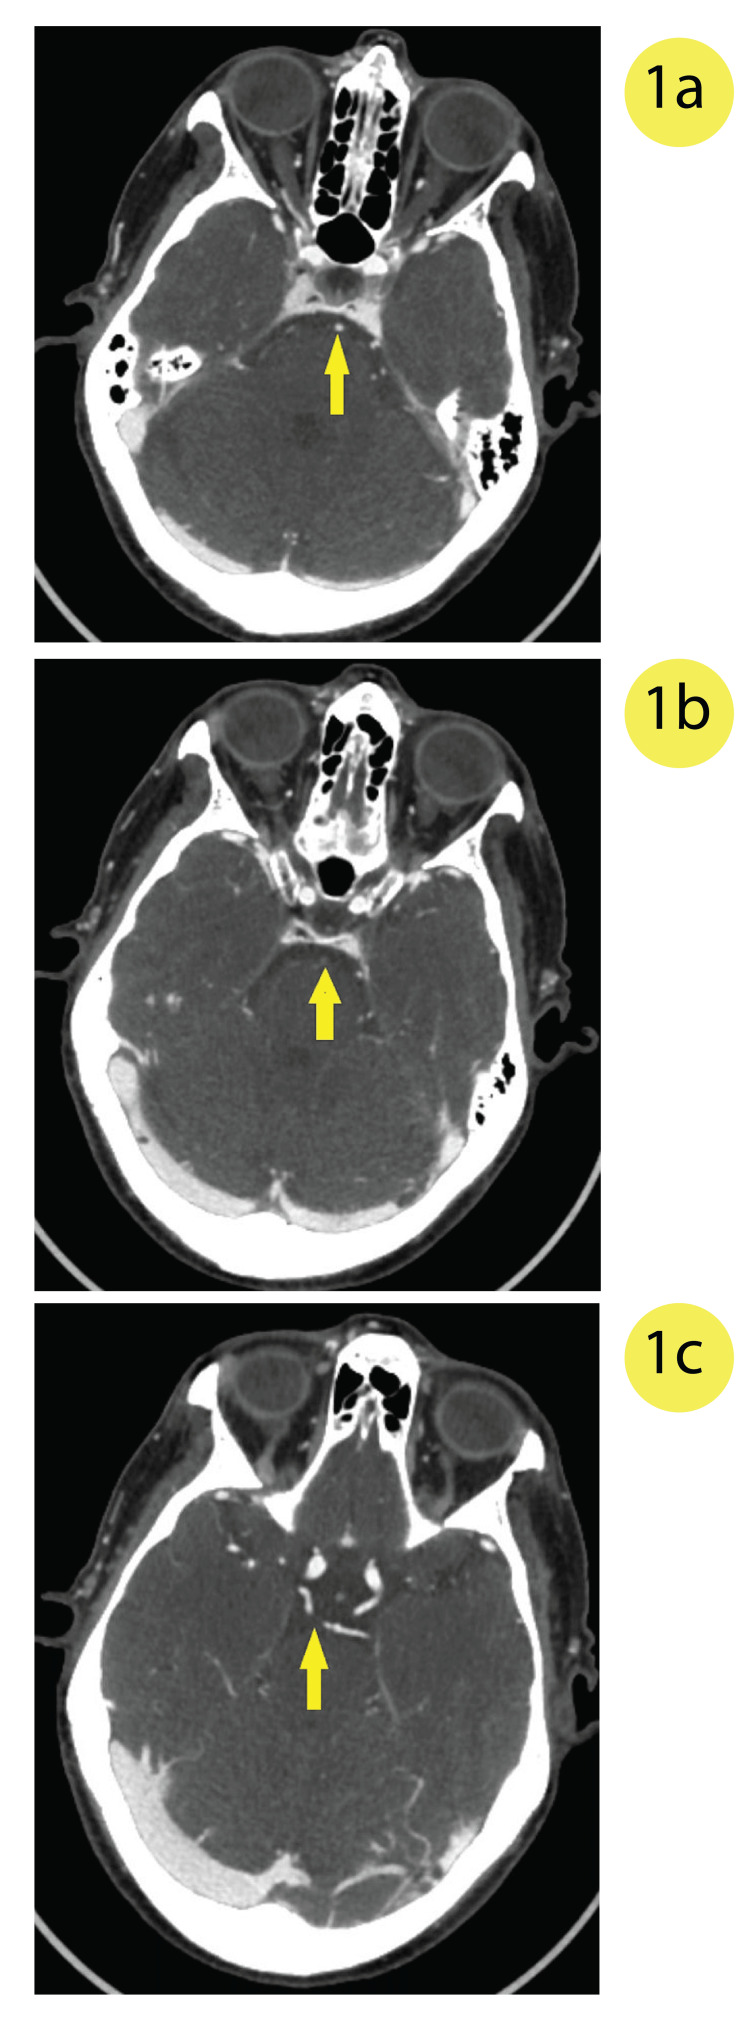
CT angiogram of the head showing a) the basilar artery just inferior to the occlusion, b) the basilar artery at the level of the occlusion, and c) reduced flow in the right superior cerebellar artery
